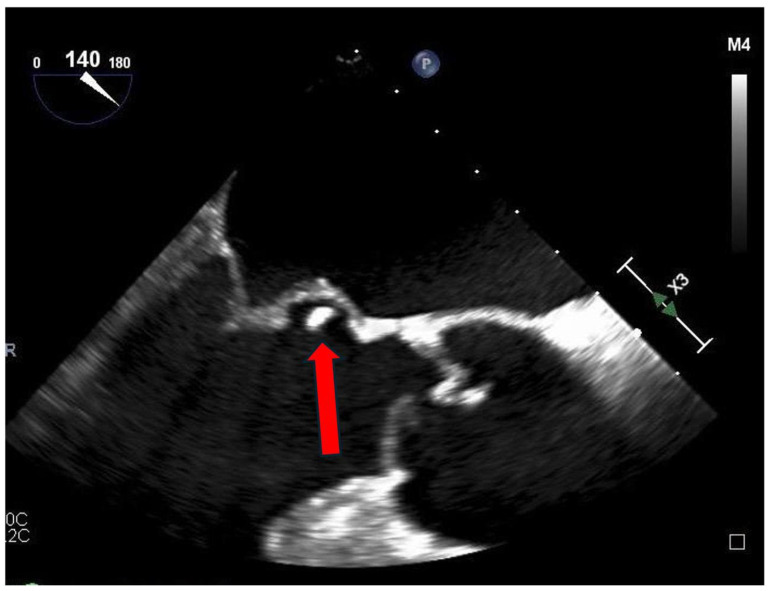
Transesophageal echocardiographic visualization of valvular vegetation. Mid-esophageal transesophageal echocardiographic long-axis view demonstrating an echogenic, irregular mass attached to the valvular apparatus, protruding into the cardiac lumen. The lesion is clearly distinguishable from adjacent valve tissue and is visualized along the line of leaflet coaptation, consistent with a mobile valvular aneurysm. The arrow indicates a mobile echogenic mass attached to the valve leaflet, consistent with valvular vegetation, a characteristic finding in infective endocarditis.
